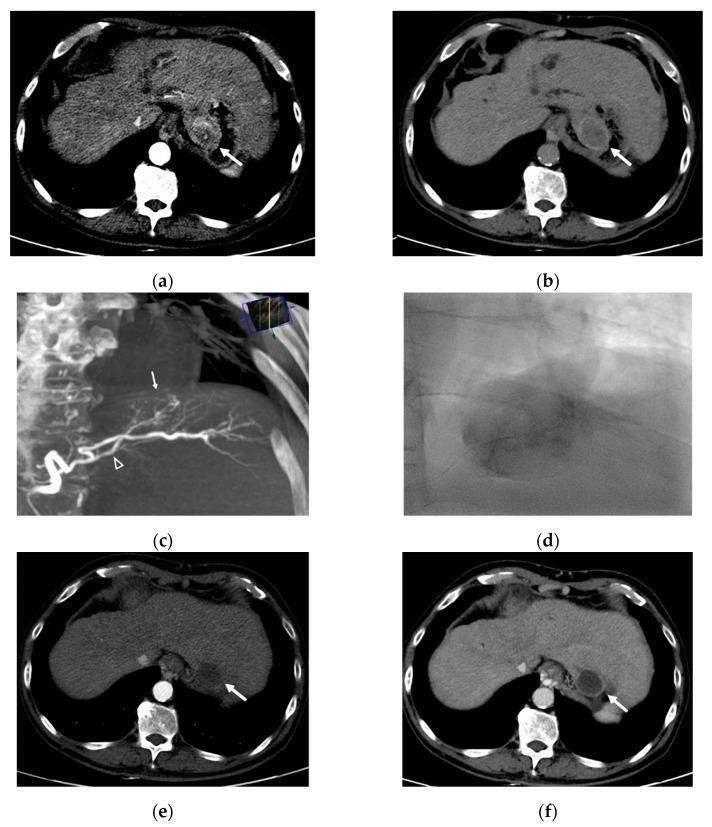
A 61 year-old man with HCC. (a) Arterial phase of dynamic CT shows a hypervascular mass measuring 4.1cm in diameter in segment 2 (arrow). (b) Delayed phase image from the same study shows classic tumor washout (arrow). (c) Cone-beam CT angiography shows a tumor blush (arrow) and precisely delineates single tumor feeder (arrowhead). (d) After selection of tumor feeder using microcatheter, DC Bead (70–150 µm) loaded with doxorubicin/nonionic contrast suspension is slowly injected. (e,f) Arterial and delayed-phase CT scan 3 months later shows no enhancement in the tumor, indicating complete response.
